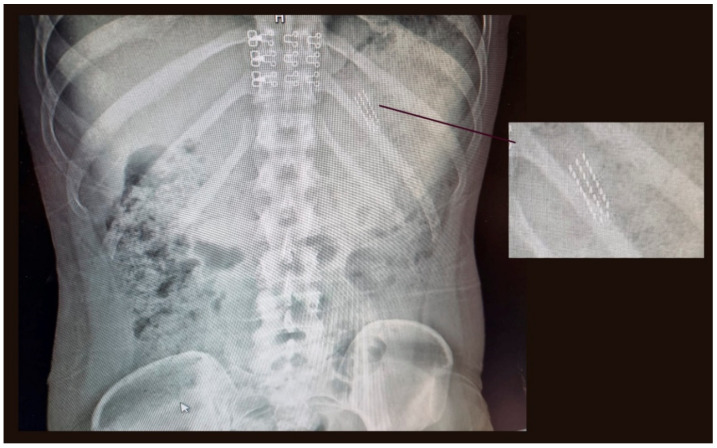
Chest and abdominal X-ray.
